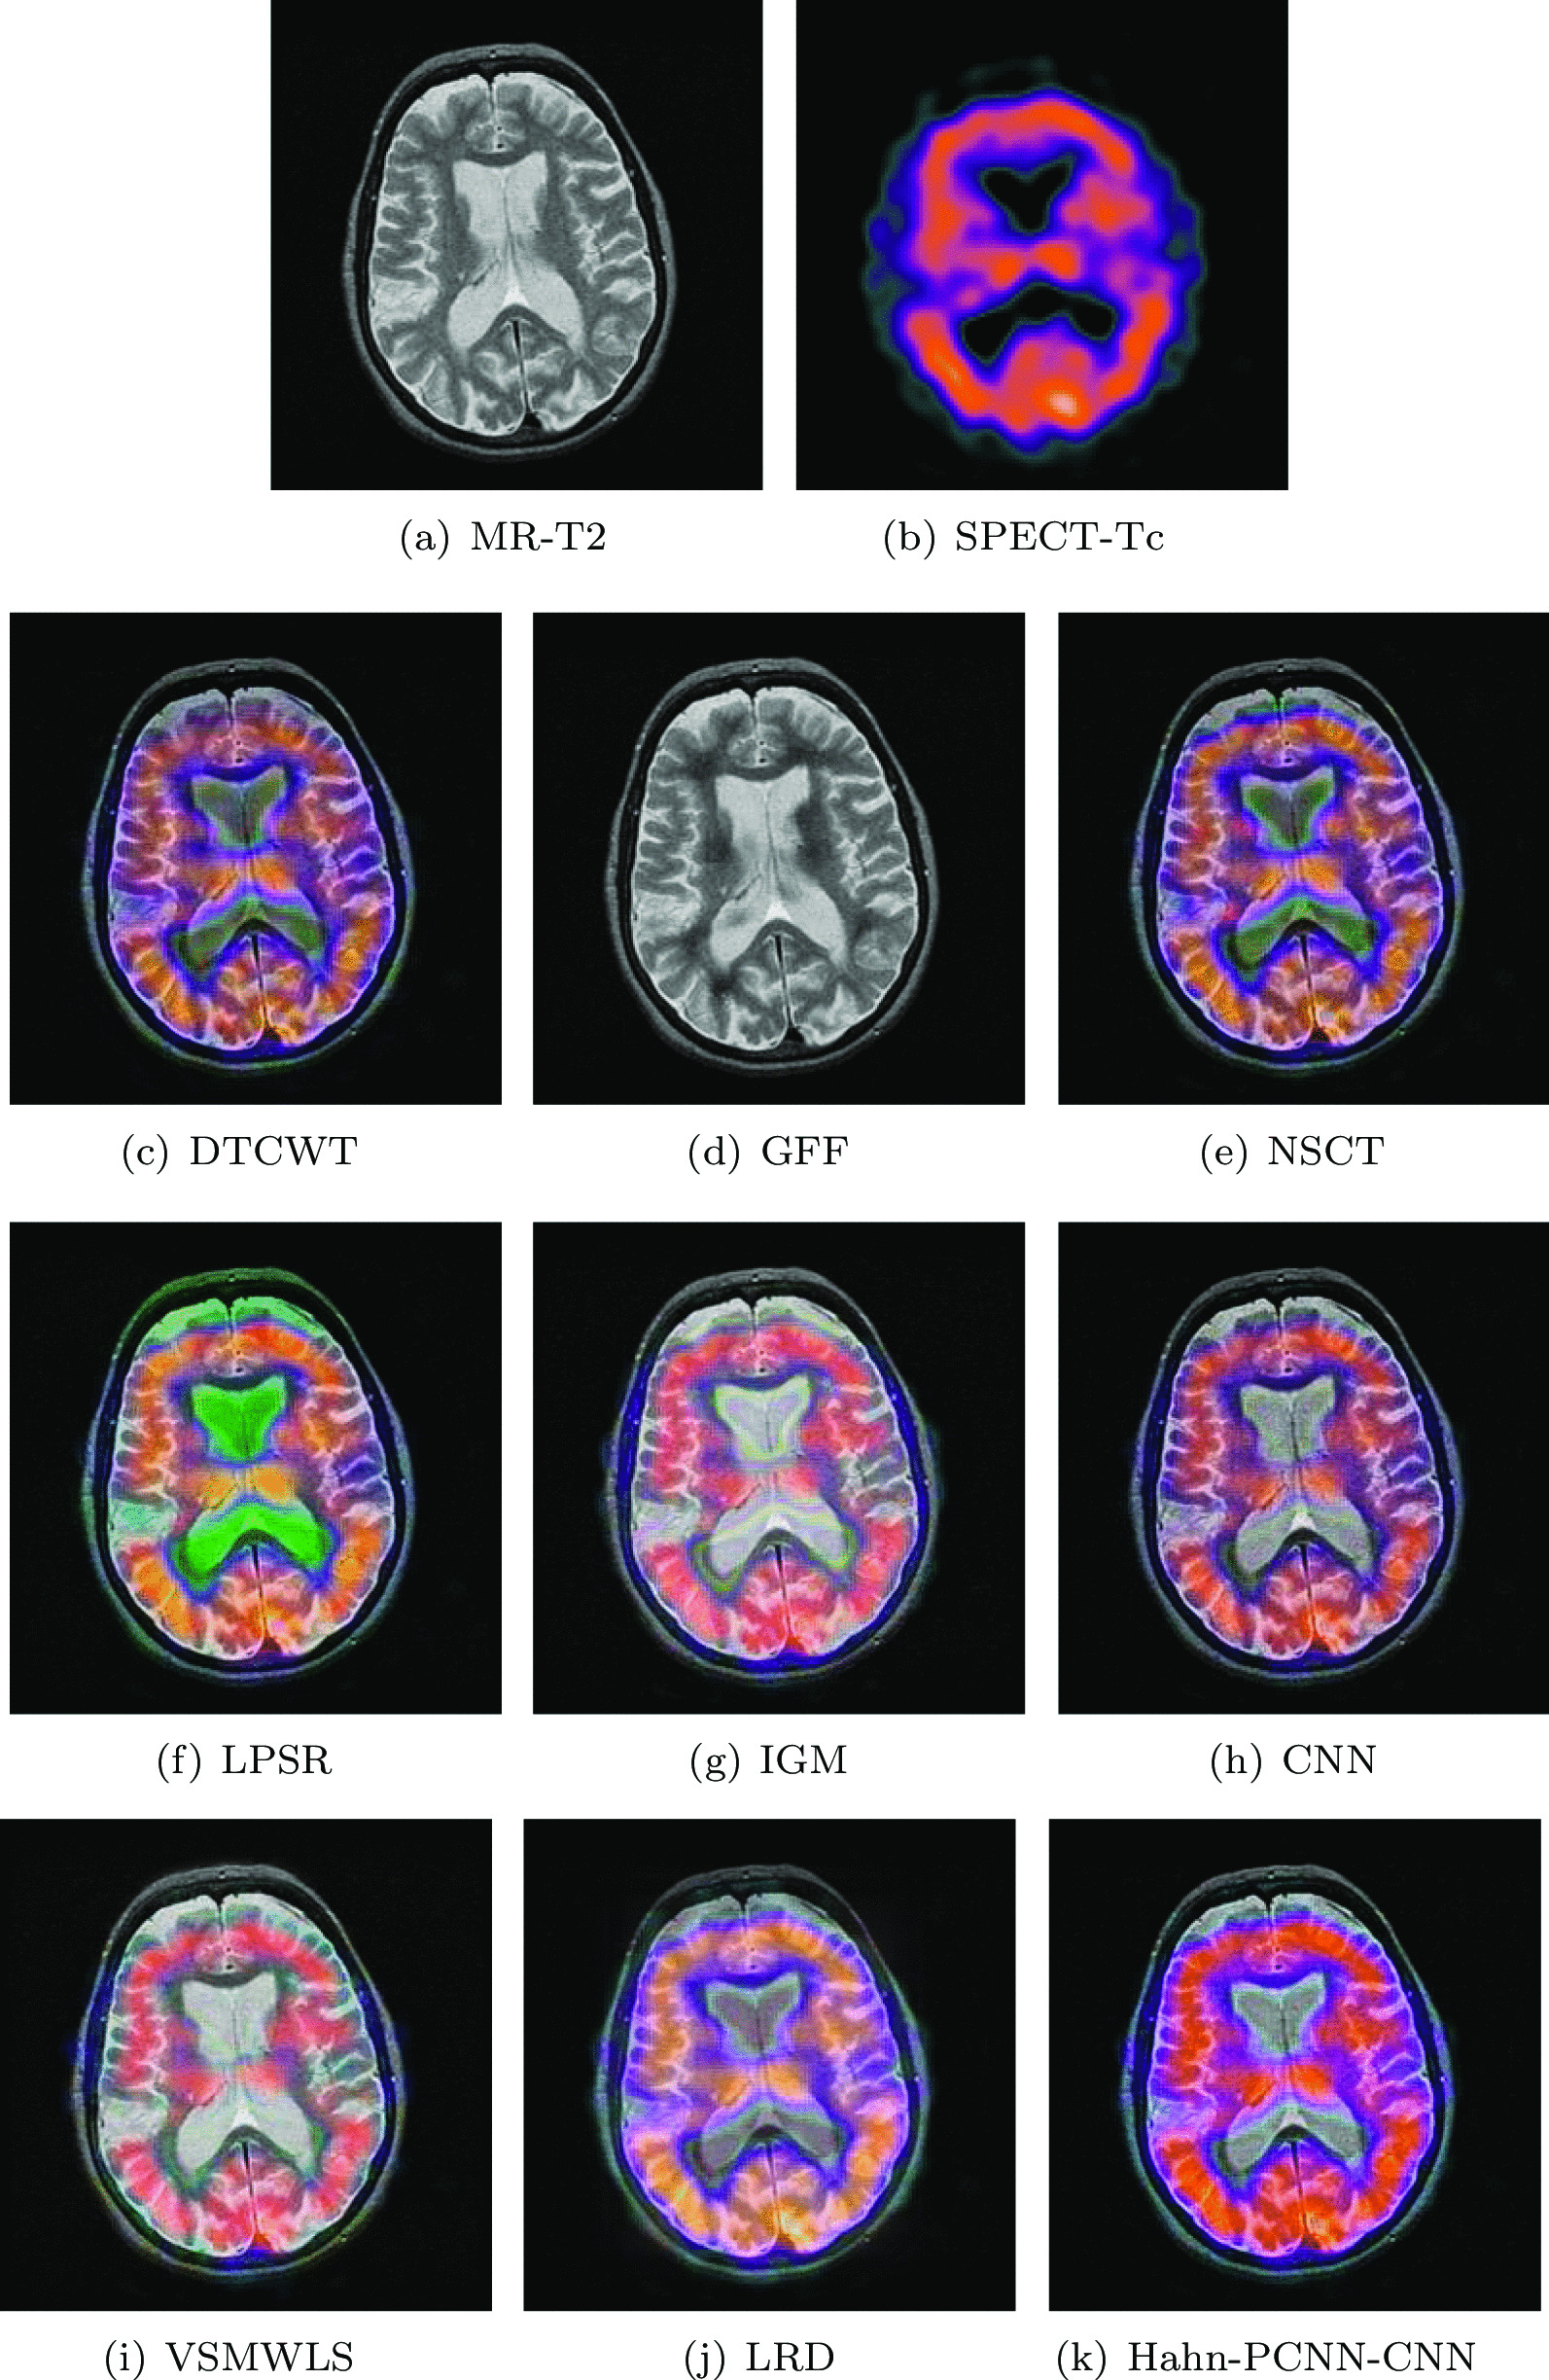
The second set of fused MRI-SPECT images from 9 methods on huntington’s disease
